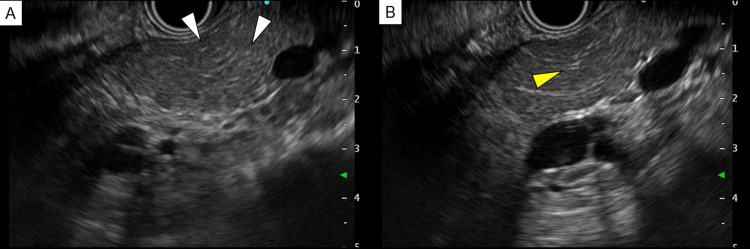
Endoscopic ultrasound-guided fine-needle aspiration Endoscopic ultrasonography-guided fine-needle aspiration showed punctate hyperechoic lesions in the pancreatic parenchyma (A: white arrow) and hyperechoic lesions at the periphery of the main pancreatic duct (B: yellow arrow).
